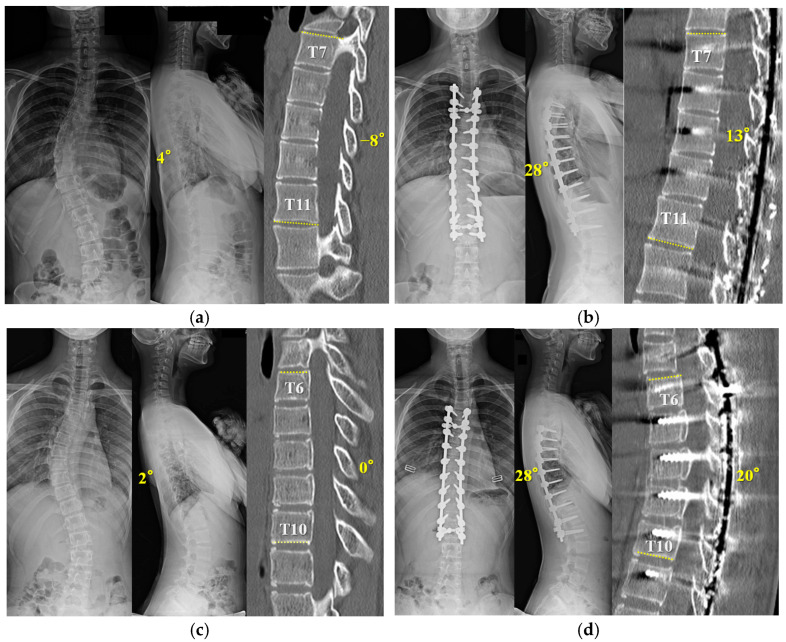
The X-ray and CT show a fifteen-year-old girl with Lenke 1A- scoliosis (a,b), and a thirteen-year-old girl with Lenke 1B- (c,d). (a) Preoperative X-ray shows a Cobb angle of 48° and 4° of TK. Apical five vertebral kyphosis is −8 degrees. (b) Postoperative X-ray shows a Cobb angle of 5°and 28° of TK after posterior fusion and correction using the outrigger. Apical five vertebral kyphosis is improved by 13 degrees. (c) Preoperative X-ray shows a Cobb angle of 51° and 2° of TK. Apical five vertebral kyphosis is 0 degrees. (d) Postoperative X-ray shows a Cobb angle of 5°and 28° of TK after posterior fusion and correction using the outrigger. Apical five vertebral kyphosis is improved by 20 degrees.
